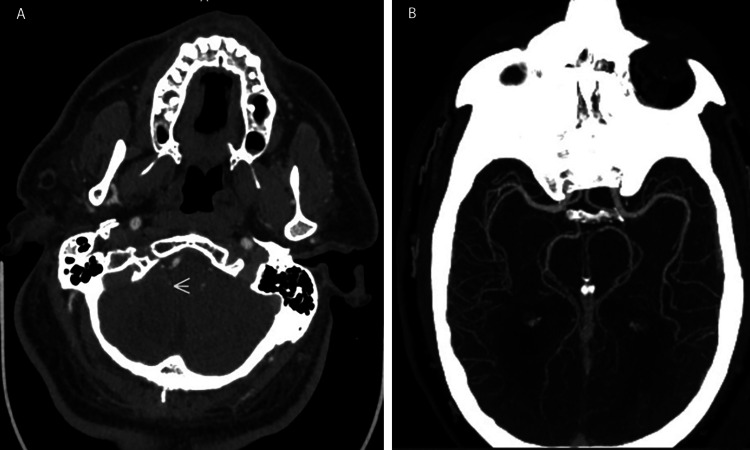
CT scans. A. CTA shows the area affected by the right proximal posterior inferior cerebellar artery occlusion (white arrows). B. AI algorithm (Viz.ai., San Francisco, CA) CTA demonstrating no large vessel occlusion.
